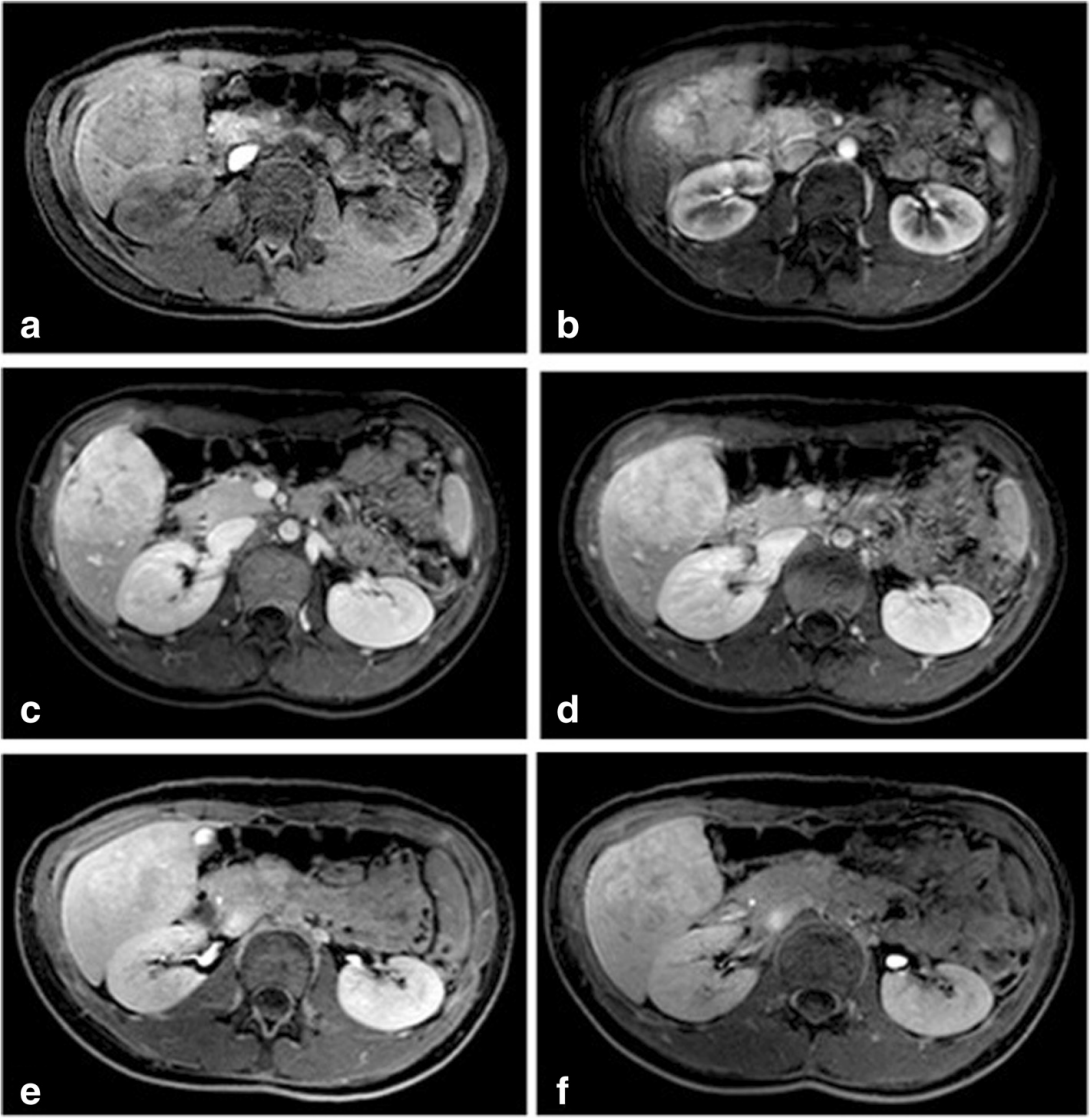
Focal nodular hyperplasia in a 14 year old female patient. The hepatic lesion is hypointense to background liver parenchyma on (a) pre-contrast T1-weighted fat-saturated sequences. There is avid enhancement of the lesion post gadobenate dimeglumine administration in (b) the arterial phase, with eventual homogenous enhancement of the lesion and central scar in the (c) portal venous and (d) equilibrium phases. The delayed (e) 30 min and (f) 45 min images show the lesion enhancing to a similar intensity as the background liver parenchyma
